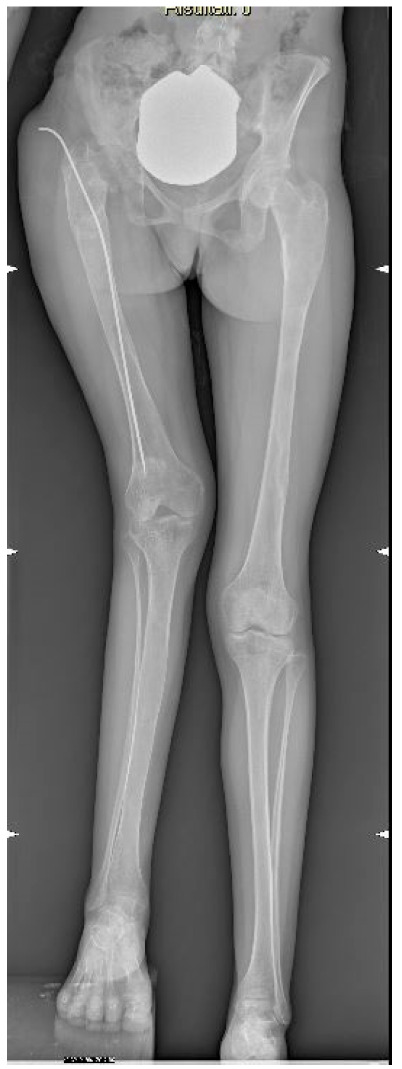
Patient X-ray: 10 cm of LLD, coxa vara and valgus knee.
